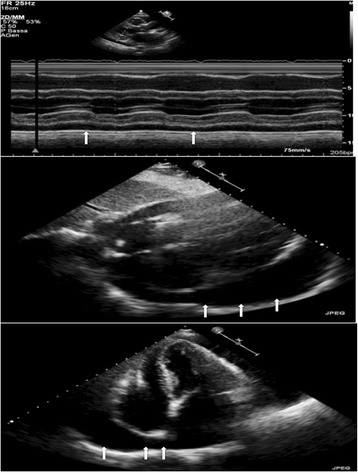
Transthoracic echocardiogram (M-Mode, subcostal four-chamber and apical four-chamber view) showing moderate pericardial effusion (arrows)
